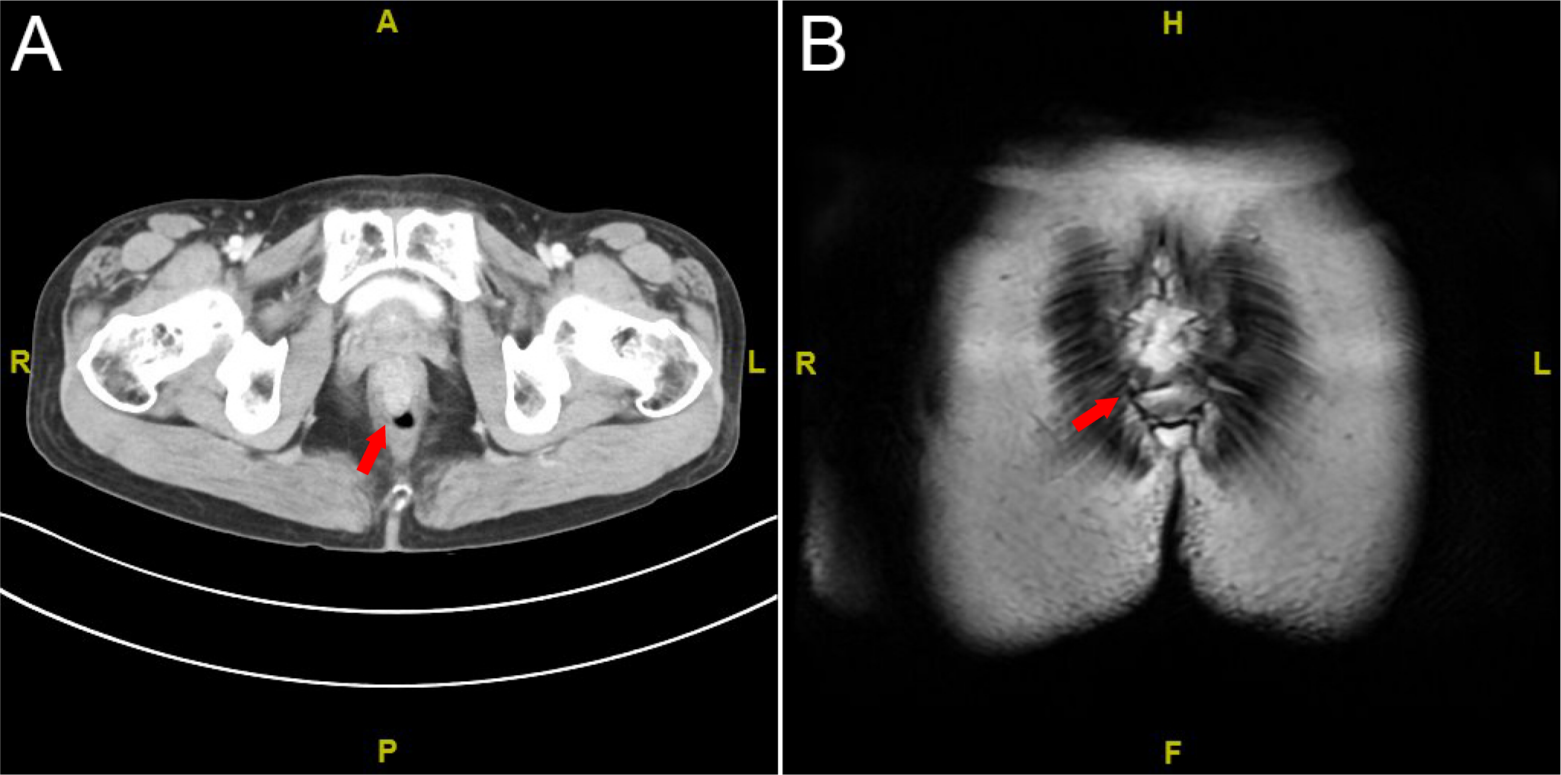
Imaging studies. (A) Contrast-enhanced abdominal CT scan; (B) pelvic MRI scan. The red arrow indicates the tumor.
